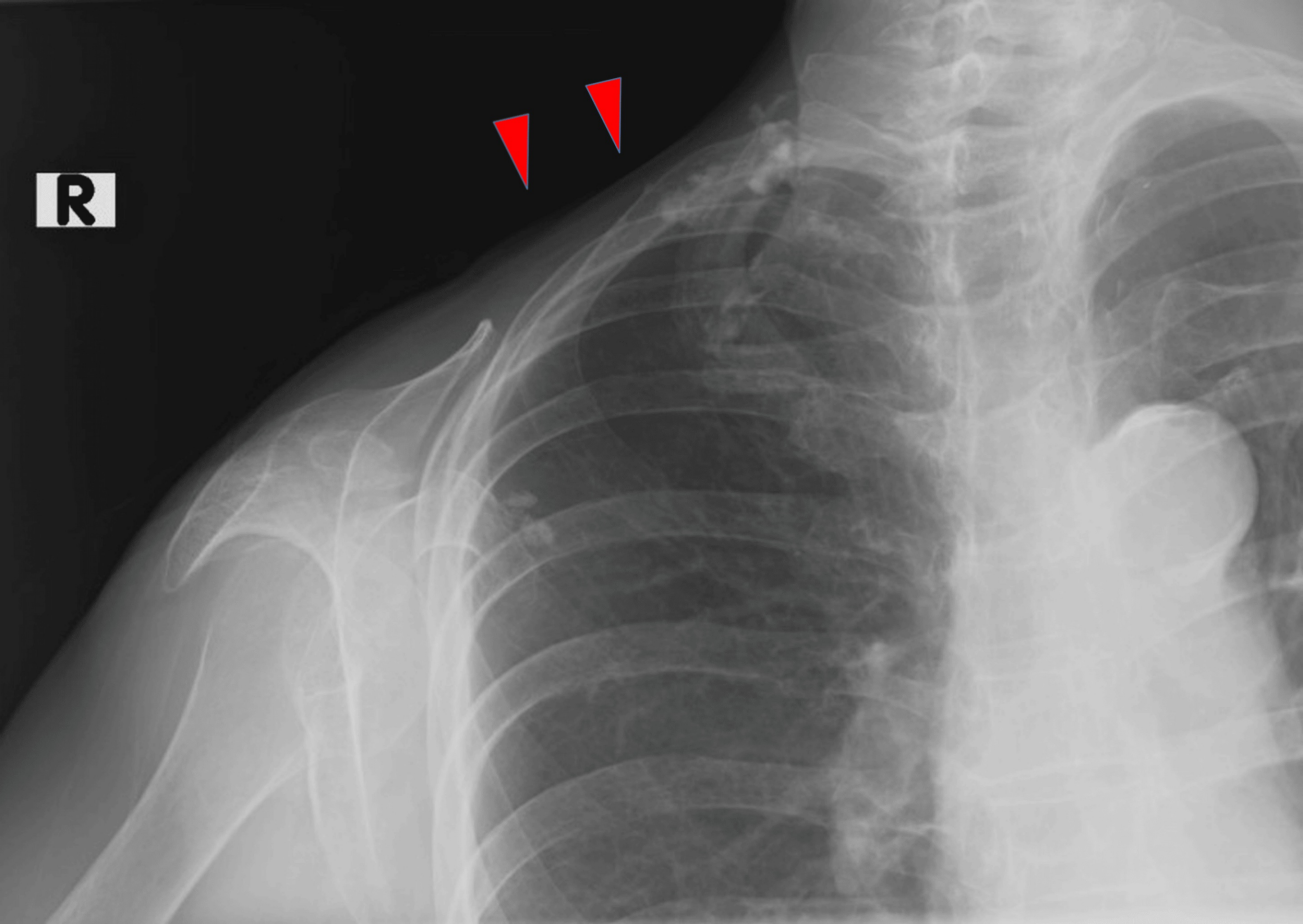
Radiograph of the right shoulder four months after debridement. The arrow points to the area of the clavicle where surgical debridement was performed.
